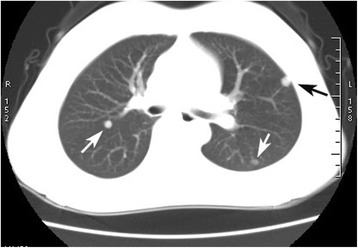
On lung window image of the chest computed tomography (CT) scan, three 3–5 mm-sized, well-defined, round nodule are seen in the bilateral pulmonary lobes, and one nodule is revealed beneath the pleura (black arrow)
